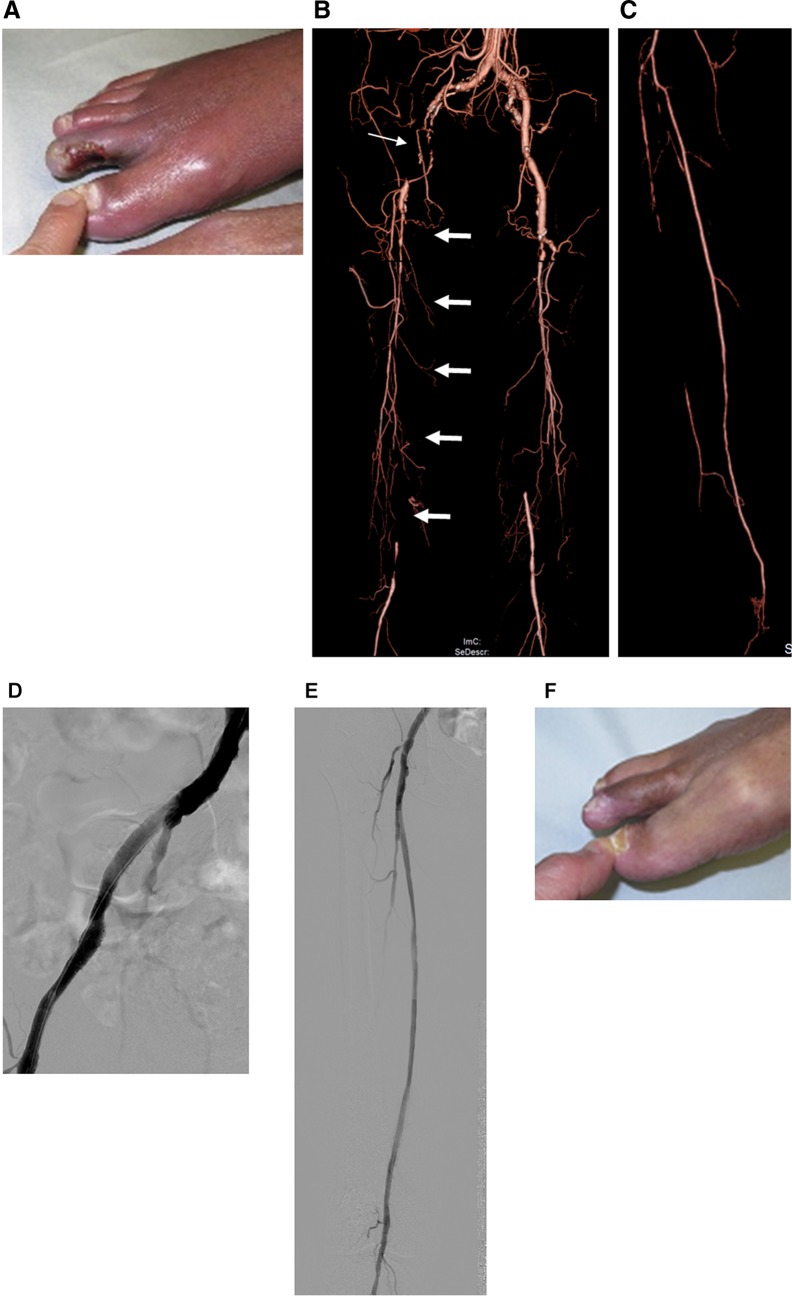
Representative case of staged procedures for multisegment disease presenting with tissue loss. a A 71-year-old male presenting with unhealing ulcer on the right second toe. Foot SPP was not measurable because of serious foot pain. b Enhanced CT revealed multisegment disease including occlusions in the right extrenal iliac (thin arrow) and femoropopliteal arteries (thick arrows). c Enhanced CT also revealed extensive occlusions in the right anterior tibial and peroneal arteries. d In the first session, right external iliac artery occlusion was successfully recanalized with stents. Given the concomitant femoropopliteal lesion complexity, contrast volume used, and procedure time, long occlusion in the femoropopliteal artery remained untreated in the session. Although rest pain significantly improved, the wound did not significantly heal with the foot SPP only 25 mmHg on the dorsum and 15 mmHg on the plantar, suggesting the need for further intervention. e In the second session, long occlusion in the right femoropopliteal artery was successfully recanalized with stents. However, given the potential of balloon-induced early reocclusion in the crural artery, the concomitant infrapopliteal artery remained untreated. f Foot SPP increased to 77 mmHg on the dorsum and 70 mmHg on the plantar, and the wound completely healed 5 months later
